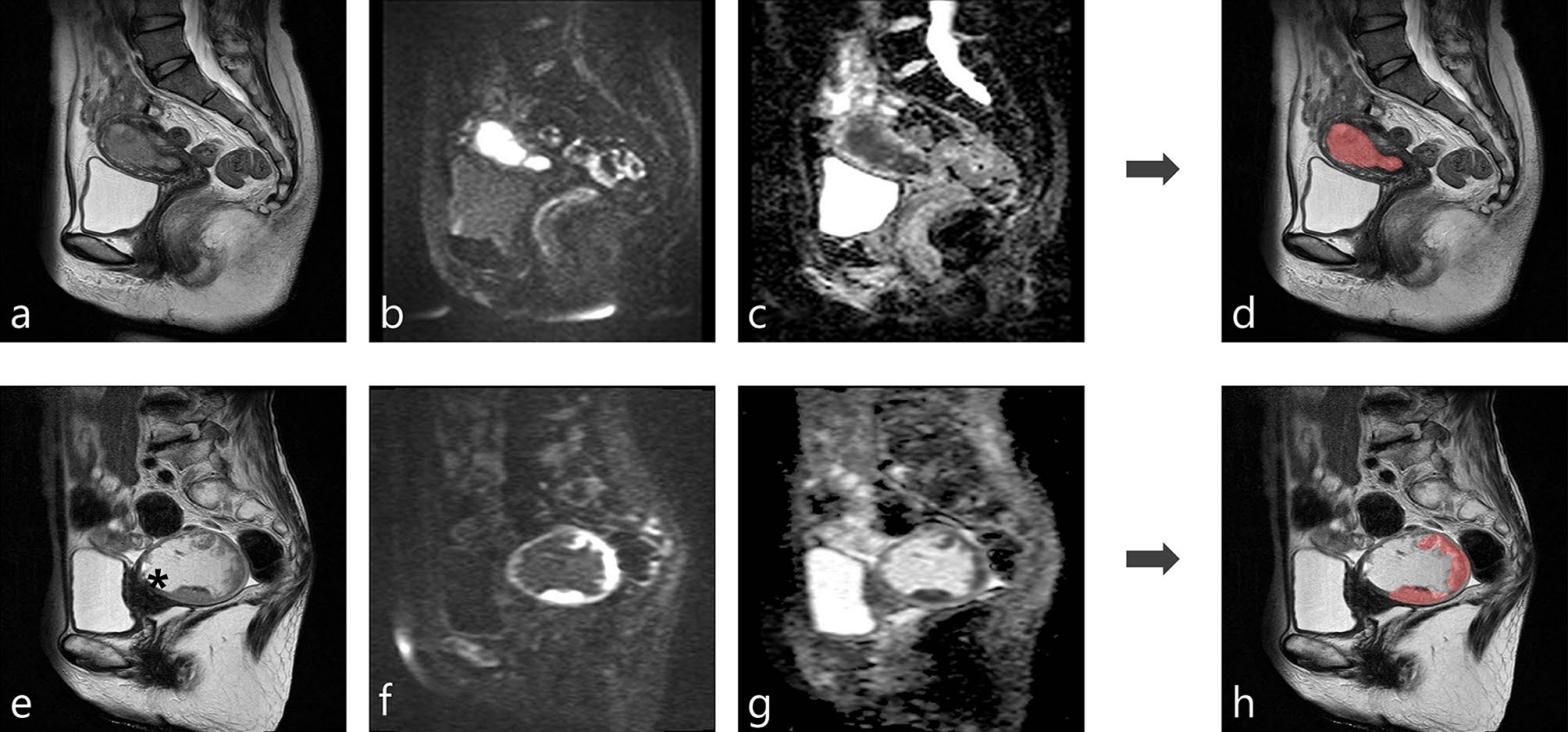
Two representative cases of automatic segmentation. (Case 1: a–d, Case 2: e–h). (a﻿, e): T2-weighted image. (b, ﻿f): Diffusion-weighted image (b = 1000 s/mm^2^). (c, ﻿g): Apparent diffusion coefficient map. (d, ﻿h): Results of automatic segmentation of endometrial cancer overlaid on a T2-weighted image. The tumor was almost perfectly segmented in case 1 (Dice similarity coefficient, 0.925). The tumor was well segmented in case 2, despite the presence of hematometra (e:*) (Dice similarity coefficient, 0.808).
